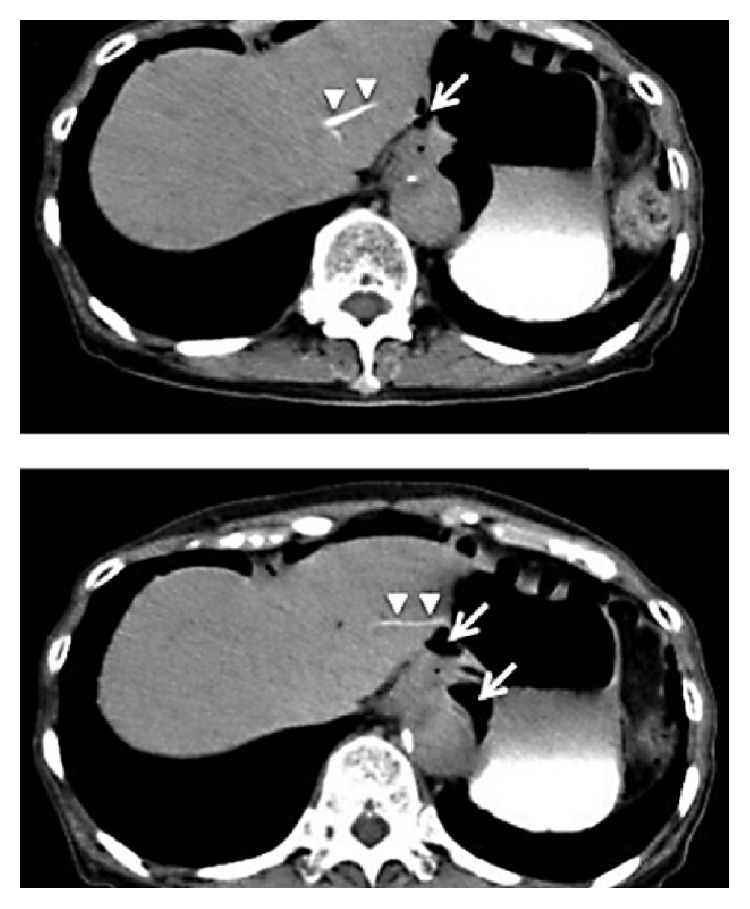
CT showing intraperitoneal air (arrow) with contrast leak from the left hepatic lobe parenchyma (arrow heads) (Kayashima et al. [9], with permission).
